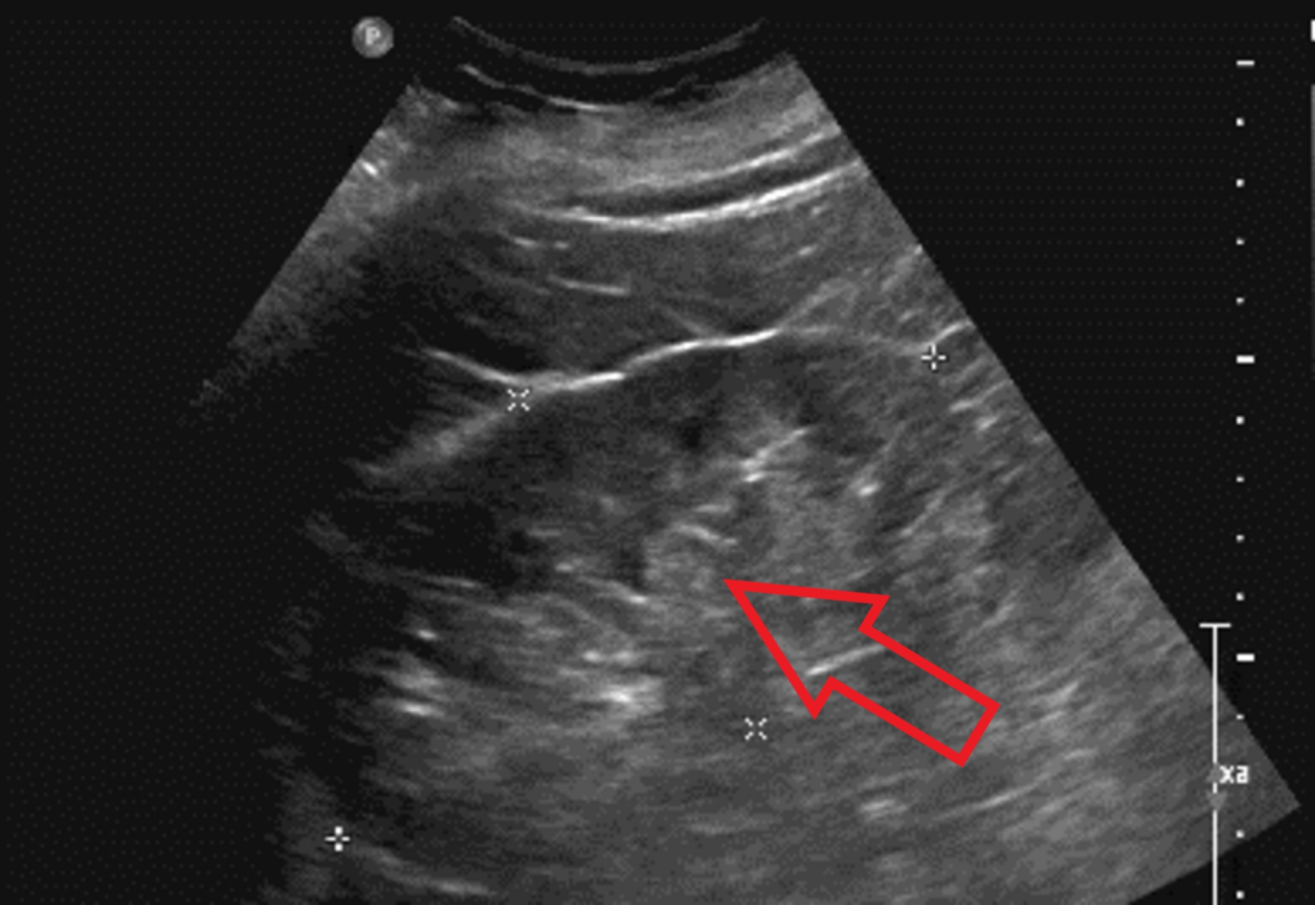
Ultrasound (US) left kidney showing echodensities (red arrow)
